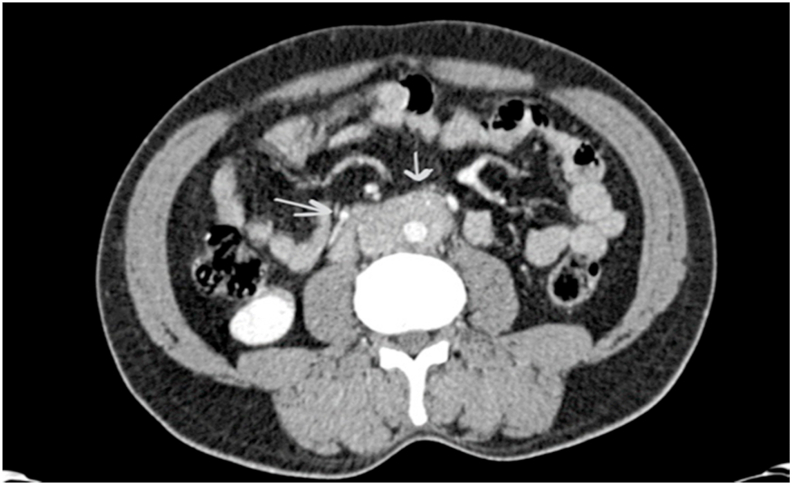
CT scan of the abdomen showing Retroperitoneal soft tissue density encasing the abdominal aorta, Inferior vena cava and common iliac arteries. (This figure does not need to be printed in color).
